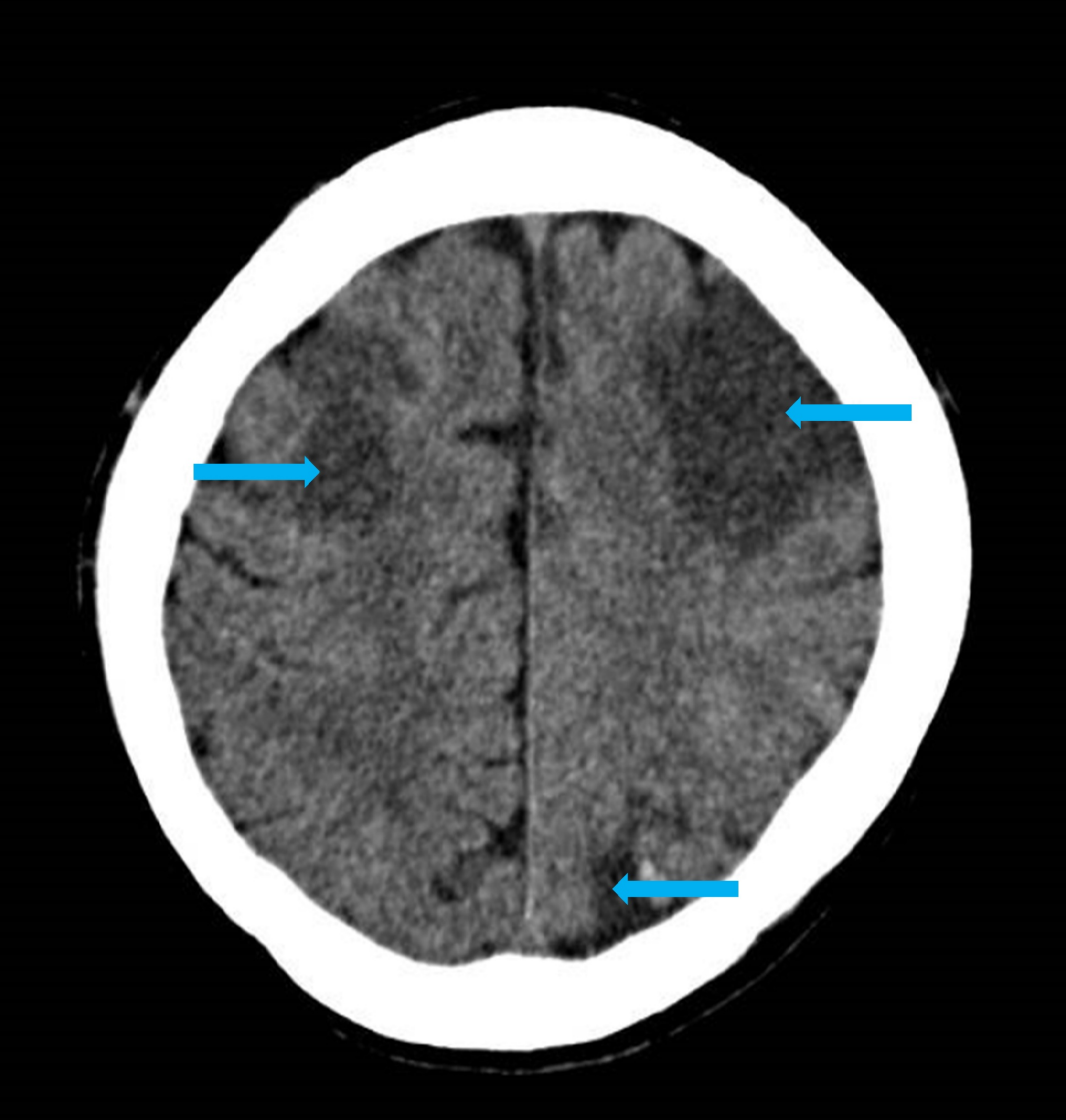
Computed tomography of the head done on day 15 after the admission Axial unenhanced computed tomography of the head shows a loss of grey-white matter differentiation and hypodensity (blue arrows) in the middle cerebral artery (MCA) territories bilaterally as well as in the left posterior parietal lobe compatible with acute infarcts
